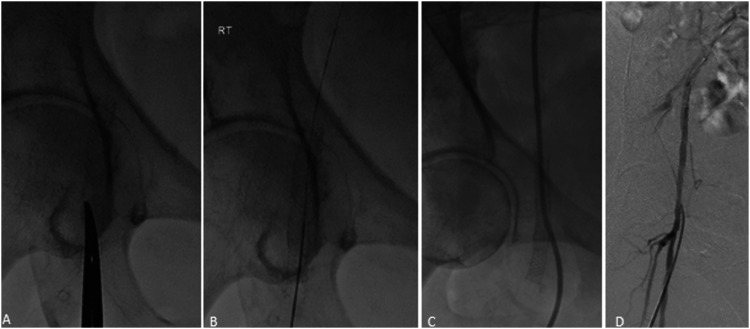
16-month follow-up angiogram. Ultrasound-guided antegrade right CFA access through the SUPERA stent with a 19-G needle (A). Insertion of a 4-Fr dilator over a Bentson wire (B, C). Post-treatment angiogram demonstrated preserved integrity of the stent with no stent-related complications (D).
